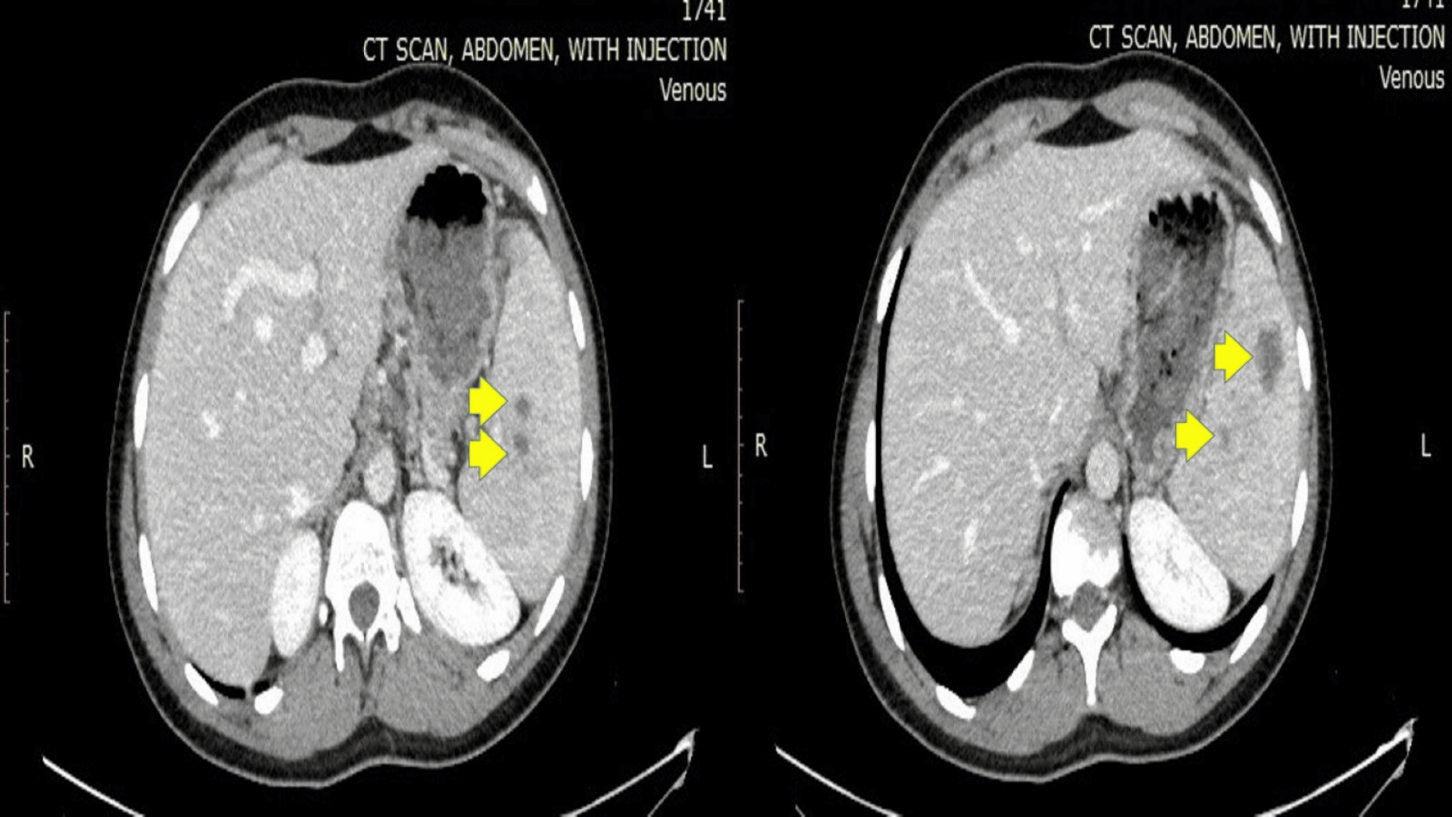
CT abdomen pelvis with IV injection shows an interval increase in the size of the hypo-enhancing splenic lesions (yellow arrows) that are likely related to the previously described splenic abscesses in the context of Crohn's disease, suggestive of an acute flare of Crohn's disease.
